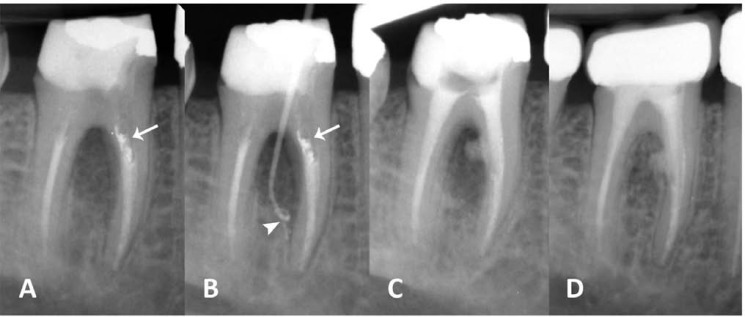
A) *Pre-operative parallel radiography of the lower first molar with an extensive furcation lesion and defective coronal restoration. Note the opaque material in the mesial root (white arrow); *B)* Diagnostic radiography after tracing the sinus tract with a gutta-percha cone that ends up within the furcal lesion (white arrow head). Again, note the opaque cement in the mesial root (white arrow); *C)* Post-operative radiograph; note the flow of CEM cement into the furcation area. The apical 1/2 of all canals are left untouched; *D) *One year follow-up radiography shows complete healing of the furcal lesion and its replacement with bone
